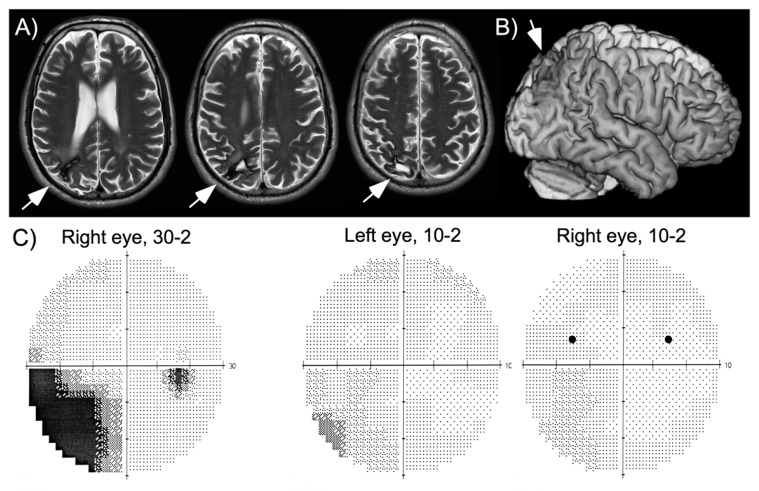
Magnetic resonance imaging (MRI) scan and visual field plot of the patient. (A) Axial slices at three different levels of the parietal lobe (images shown according to radiological convention, left is on the right). (B) Three-dimensional reconstruction of the patient’s brain, showing the small lesion in the superior parietal cortex. (C) Visual field plots (Humphrey Perimeter; 30-2 plot: sensitivity tested up to 30 degrees into the left and right hemifield; 10-2 plots: sensitivity tested for the central 10 degrees; inter-stimulus distance was always two degrees). Testing of the sensitivity up to 30 degrees in the periphery shows incomplete left lower quadrantanopia. More detailed testing of the central 10 degrees shows intact central visual fields. The two black dots indicate positions where targets were presented.
